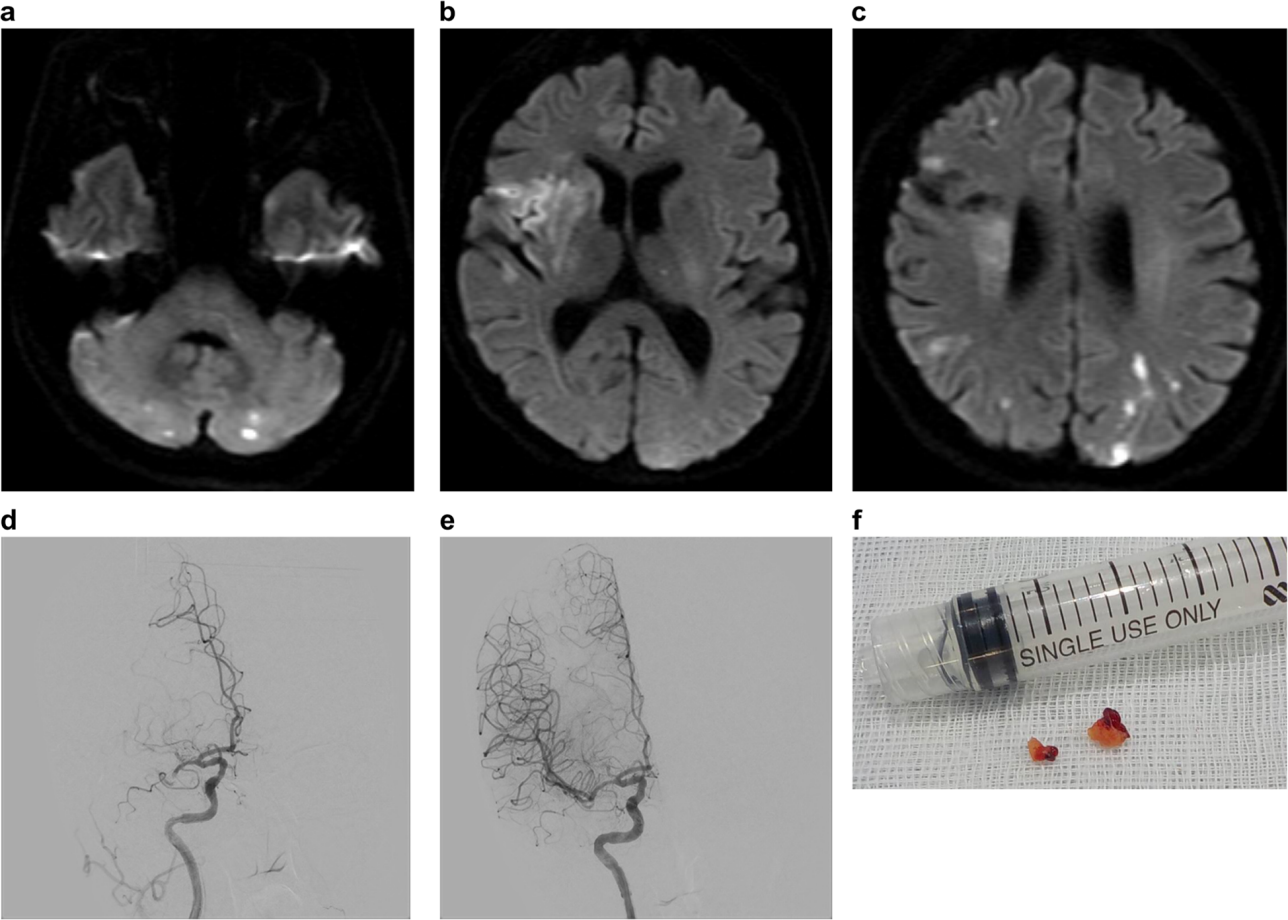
Brain imaging and thrombus collected on the day of the development of cerebral infarctions a-c Brain magnetic resonance imaging shows bilateral multiple cerebral infarctions indicating right middle cerebral artery occlusion. d, e Right internal carotid artery angiography shows right cerebral artery occlusion, and thus, we performed endovascular thrombectomy. f The collected thrombus
